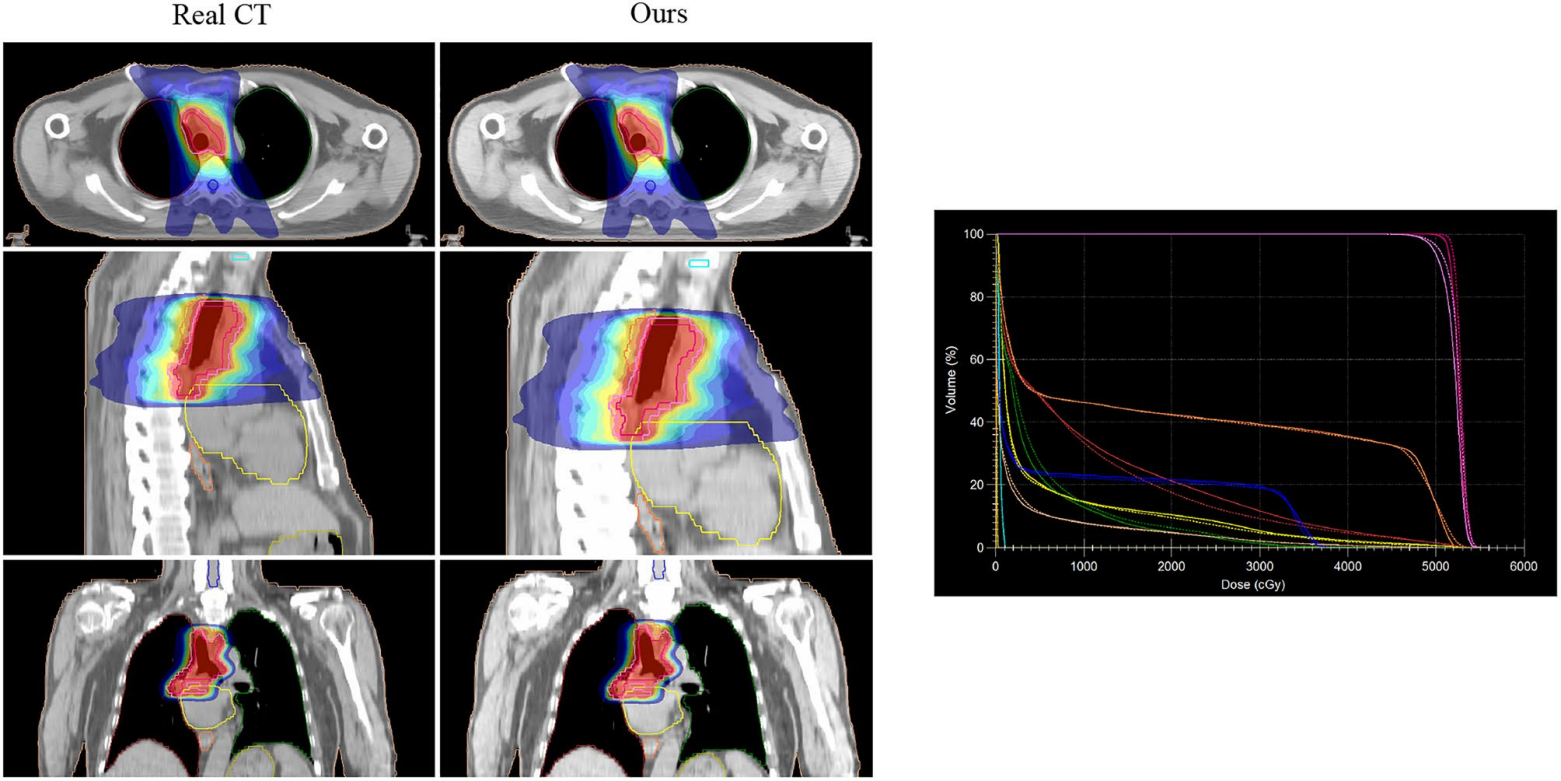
3D reconstruction visualization of the Chest dataset. From top to bottom, representing axial, sagittal and coronal planes(left). Patient DVH differences, where the solid line is Real CT and the dashed line is sCT (right).
